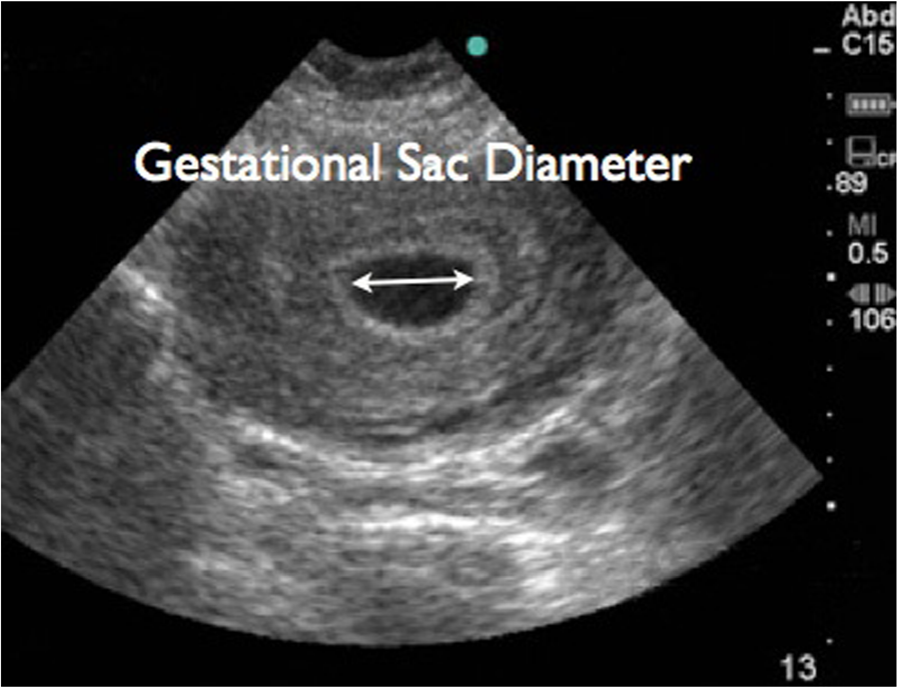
Trans-abdominal ultrasound image showing measurement of gestational sac diameter.
